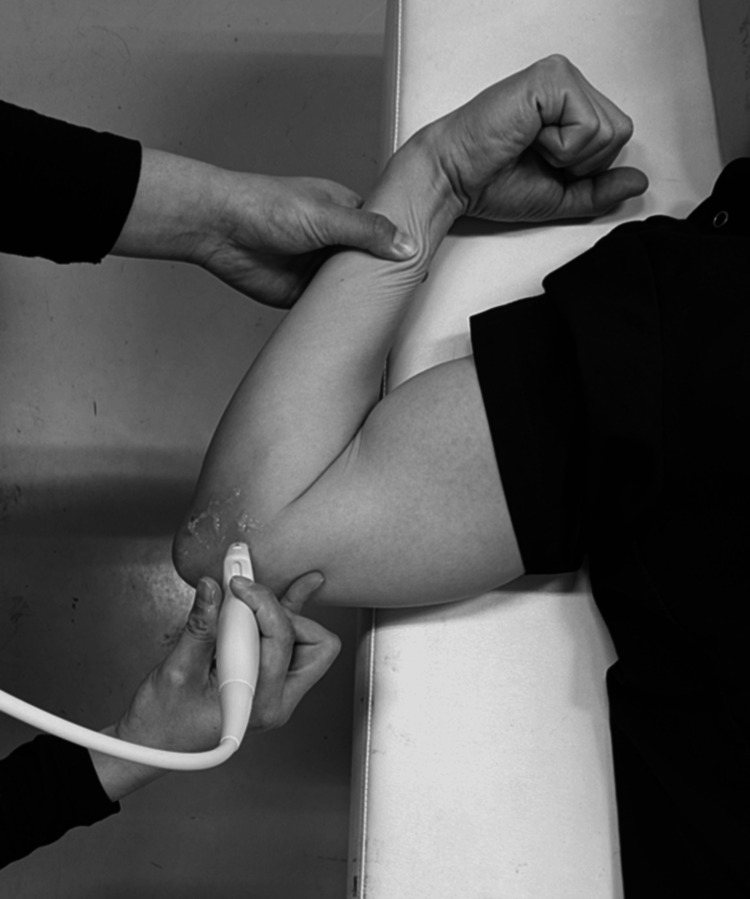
The position of the ultrasound probe during the ulnar nerve instability (UNI) evaluation
